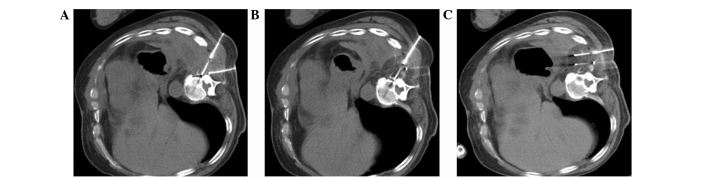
Lung cancer with rib and vertebral metastasis and bone destruction, during the ablation procedure. CT scans showing (A) the insertion of cryoprobes into metastatic lesions and (B) the monitoring of the area of ablation, and (C) ensuring the ablation area completely covers the lesion. CT, computed tomography.
